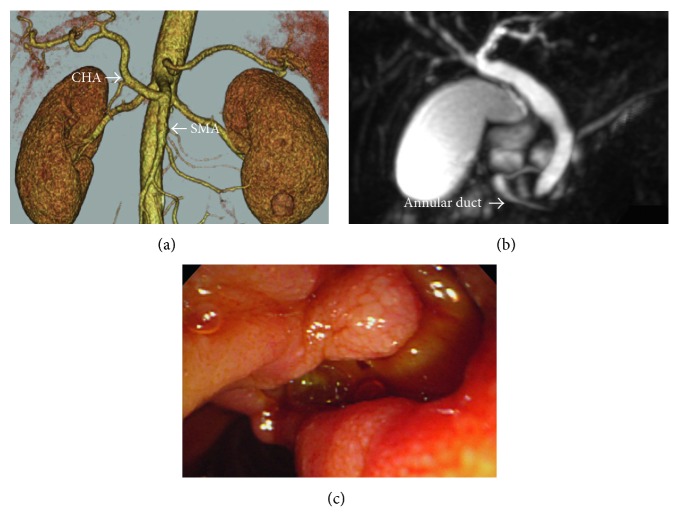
Preoperative image findings. (a) Three-dimensional computed tomography scan of the abdomen reveals the replaced common hepatic artery arising from the superior mesenteric artery. (b) Magnetic resonance cholangiopancreatogram shows the annular duct, but no relevant tumor or dilation of the common bile duct and the pancreatic duct. (c) Esophagogastroduodenoscopy shows an ulcer at the ampulla of Vater, and the biopsy results of the ulcer indicate adenocarcinoma. Cannulation of the papilla region could not be performed because of direct invasion of the tumor.
